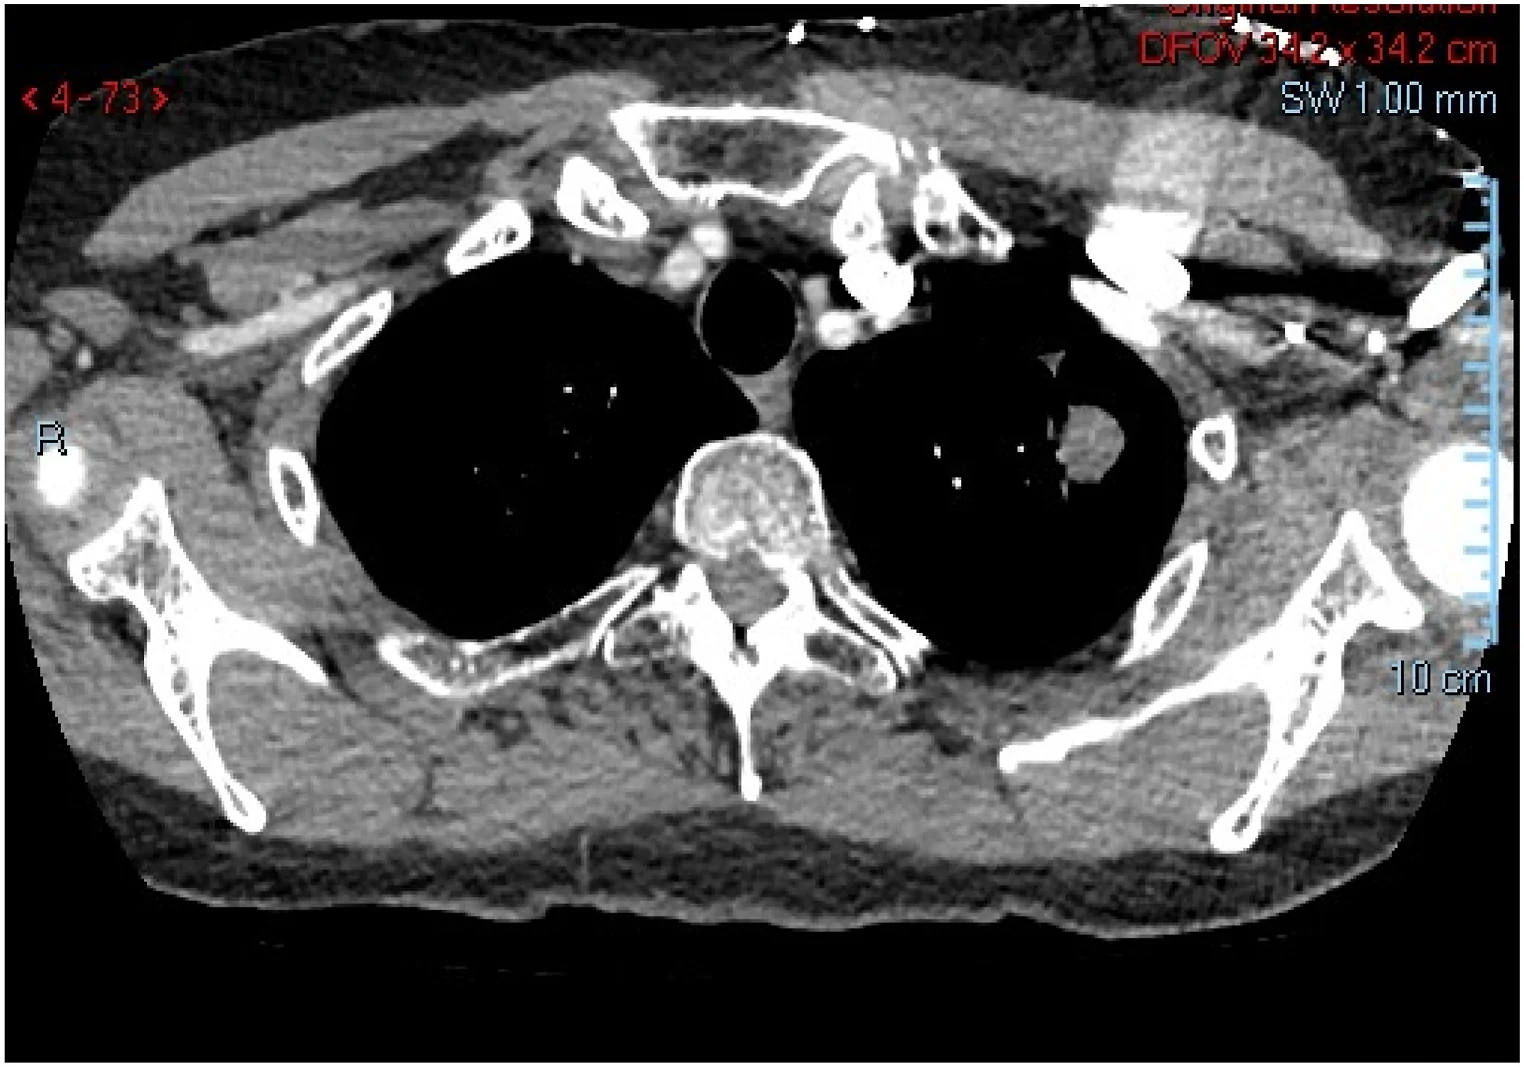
Angio-CT Thorax with 3D reconstructions for surgical planning (January 7, 2026).
